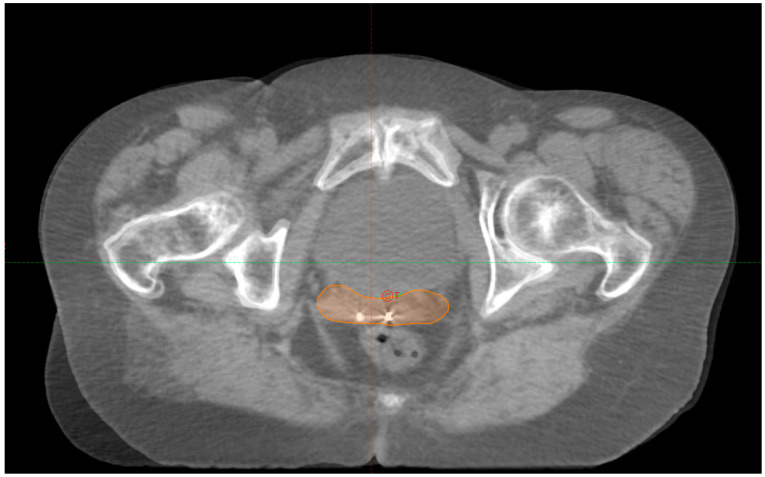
An example of the IGRT technique applied in this observational study (pt4—fraction 2); pCT imaging with the vaginal CTV segmented registered with daily CBCT based on the alignment of the implanted FMs.
